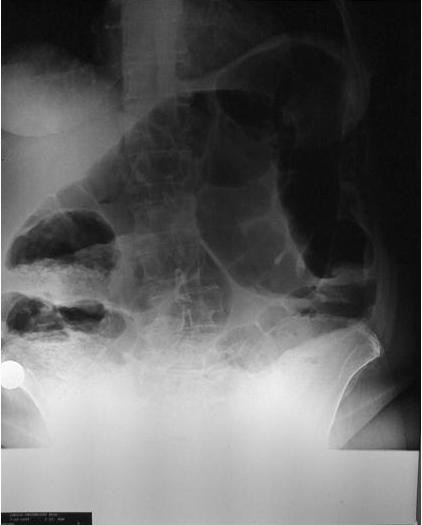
Erect abdominal X-Ray of the patient at presentation.
